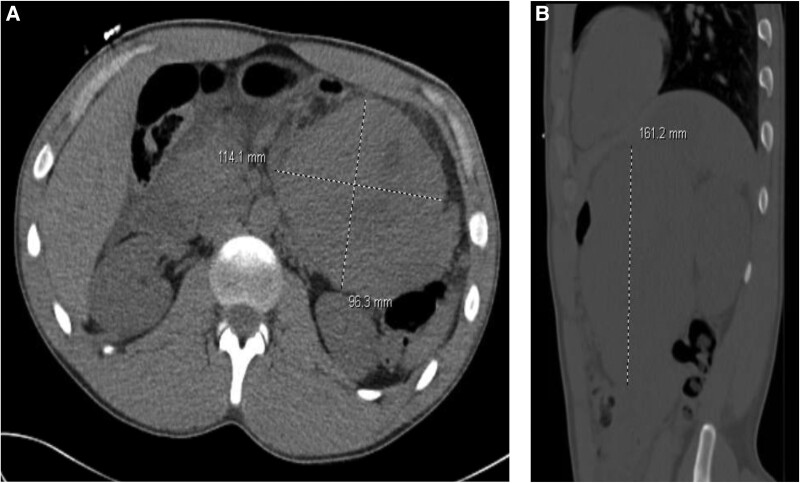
CT of the abdomen and pelvis without IV contrast demonstrating large left retroperitoneal mass inseparable from the pancreatic body and tail. Mass appears to be generally solid with slight hypodensity seen centrally. Mass compresses the stomach, displaced anteriorly. (A) Transverse view of retroperitoneal mass. (B) Sagittal view of retroperitoneal mass.
